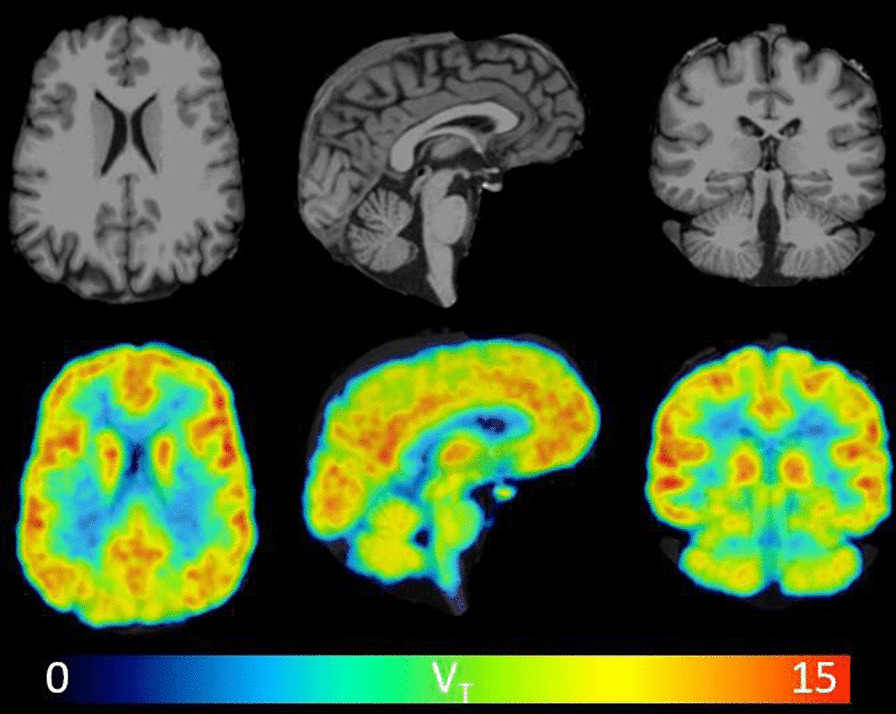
Visual inspection of ^11^C-CPPC total distribution volume (*V*_T_) values across the human brain reveals thalamus as one of the regions of highest binding, and relatively lower binding in cerebellar cortex. Parametric *V*_T_ images and MRI images in three views are shown from a representative healthy participant. *V*_T_ was estimated from 90-min data using Logan graphical analysis with the metabolite-corrected arterial input function. *V*_T_ is in units of mL cm^−3^. The *V*_T_ image was filtered with a 2 mm FWHM 3D Gaussian to reduce noise
